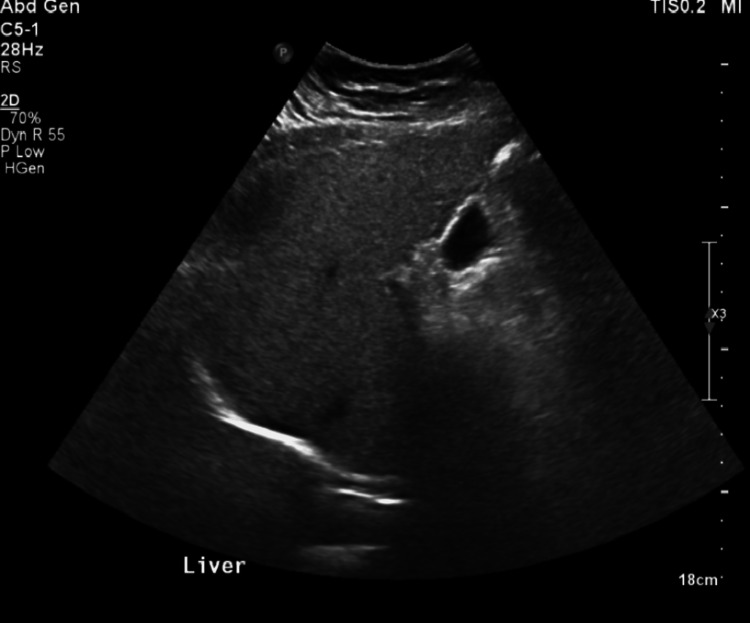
An abdominal ultrasound (US) showing mild diffuse fatty infiltration and a slight coarse echo pattern.
